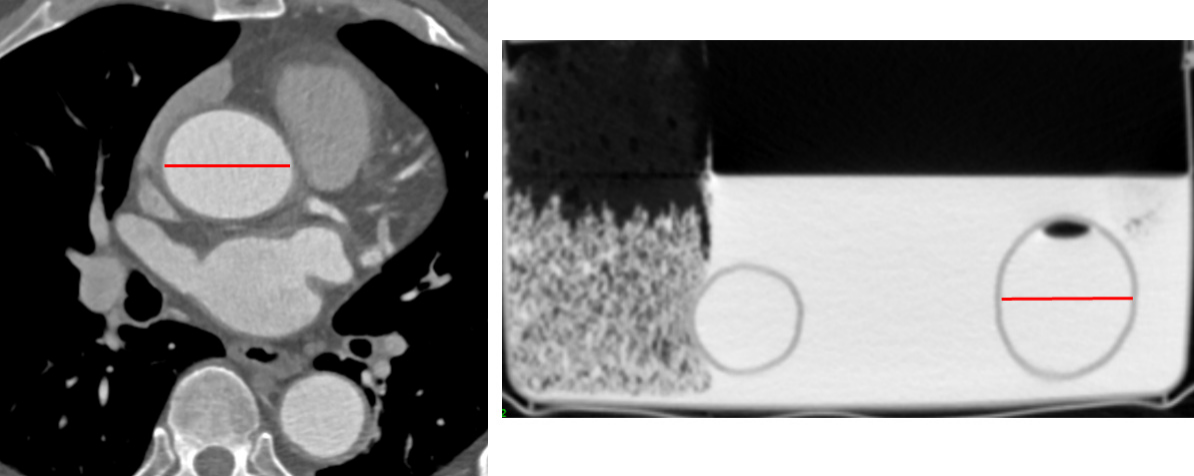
(Left) Measuring the transverse diameter of the ascending aorta in the patient's CT scan using the line tool in Analyze software. (Right) Measuring the transverse diameter of the descending aorta in the contrast‐enhanced CT scan of the 3D printed model using the line tool in Analyze software.
